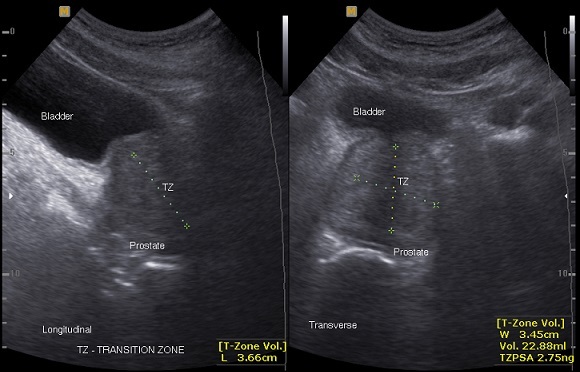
Transabdominal ultrasound of the prostate showing measurement of the transitional zone
